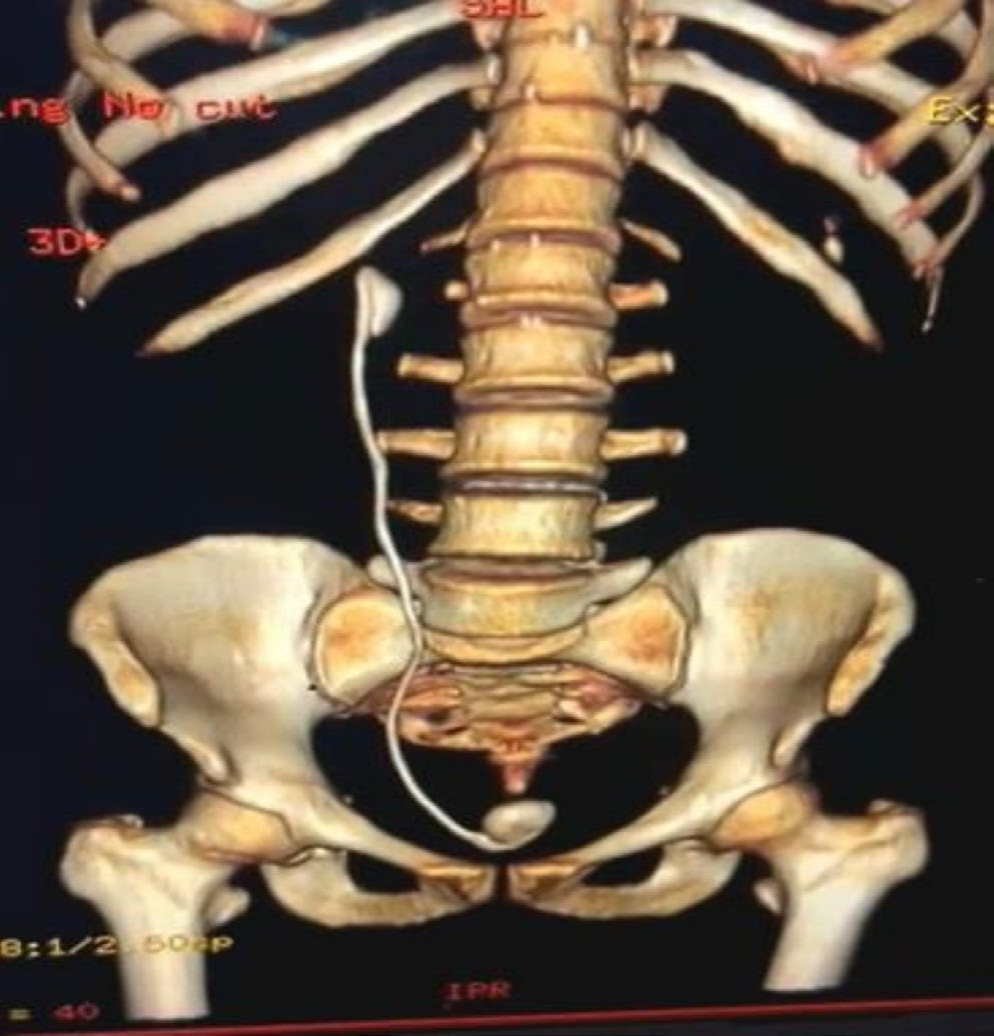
Computer tomography (CT) with 3D reconstruction.
